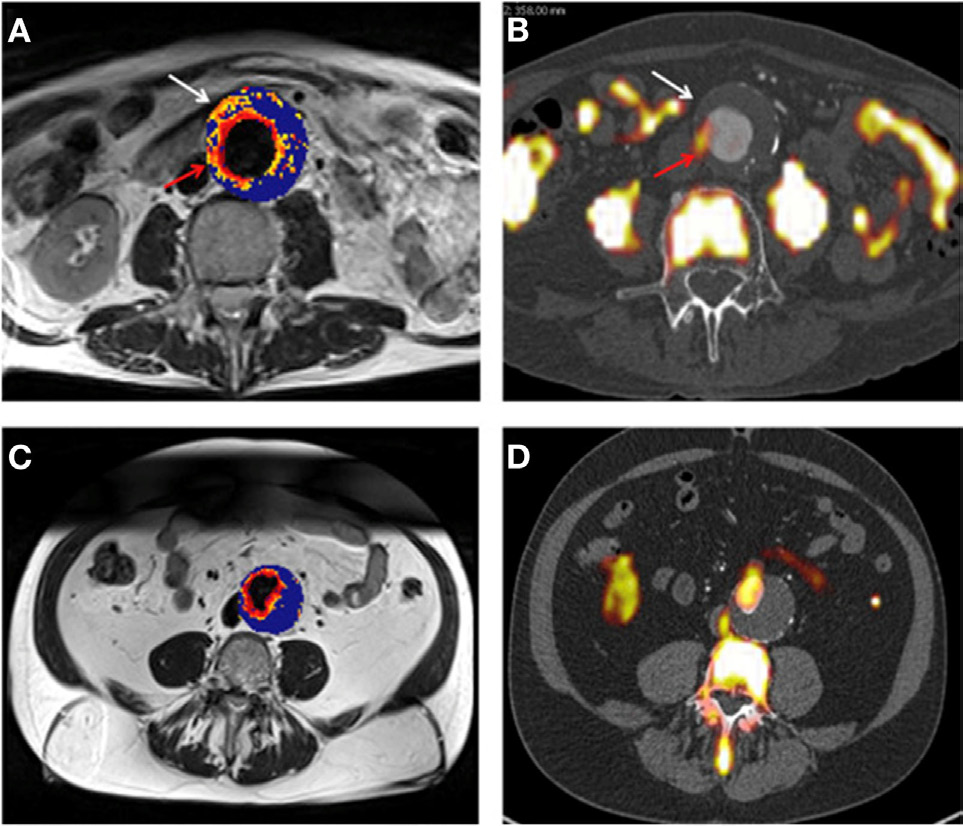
(A,B) Representative magnetic resonance imaging (MRI) (A) and fused positron emission tomography-computed tomography (PET-CT) (B) scans from the same patient with an abdominal aortic aneurysm (AAA). Ultrasmall superparamagnetic particles of iron oxide (USPIO) uptake, defined by percentage change in $T_{2}^{*}$ are demonstrated using a color scale. Changes in $T_{2}^{*}$ value over the threshold (59%) are presented on a graduated (yellow-red) color scale and data below the threshold appears blue. Corresponding 18F-fludeoxyglucose (FDG) activity (red arrow) is shown (B). Differences in the location of regions of uptake between the techniques are apparent, as marked by the white arrow. Panels (C,D) are corresponding MRI and fused PET-CT slices from the same patient who had no USPIO or 18F-FDG uptake in the wall of the AAA, with uptake limited to the peri-luminal area. Reprinted from McBride et al. (34), under the terms of the Creative Commons Attribution-Non-Commercial-No Derivatives License (CC BY-NC ND).
